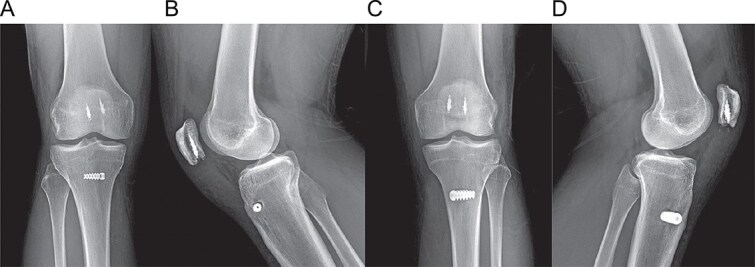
Postoperative radiographs at 1-year follow-up. (A, B) Anteroposterior and lateral views of the right knee. (C, D) Anteroposterior and lateral views of the left knee, showing stable fixation and maintained patellar height.
